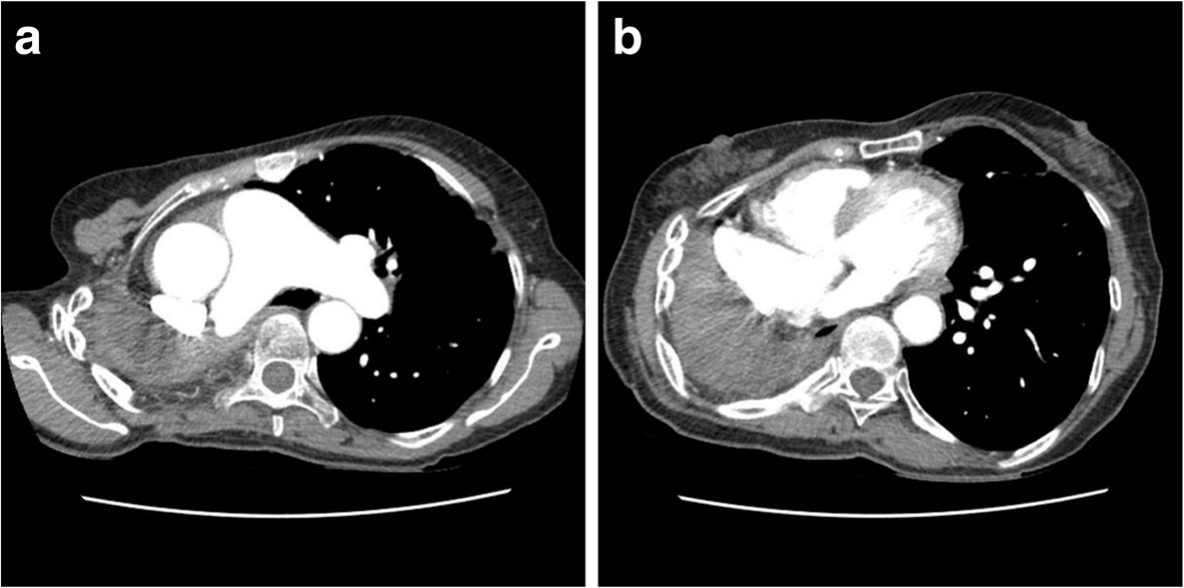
Follow up chest computed tomographic angiography showing the complete resolution of the previously noted thrombus within the right pulmonary aretry stump (a) and pulmonary embolism in left lower segmental pulmonary artery (b)
